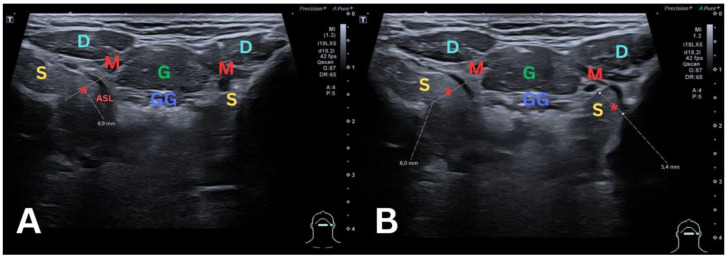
To clarify the changes, an ultrasound examination was performed. In the first image (A), the ultrasound examination, performed with a Canon Aplio i800 device, reveals a defect in the right mylohyoid muscle (M) measuring approximately 9 mm. This defect is associated with a protrusion of the right sublingual salivary gland (S), along with a protrusion of the right sublingual artery (ASL) through the muscle defect (*). In the second image (B), there is an observation of a defect (*) in the left mylohyoid muscle (M) measuring approximately 5 mm, accompanied by a small protrusion of the left sublingual salivary gland (S). These findings indicate significant anatomical changes in the sublingual region, highlighting the presence of muscle defects and glandular protrusions on both sides, which confirmed the diagnosis. S—sublingual gland; M—mylohyoid muscle; *—mylohyoid muscle defect; GG—genioglossus muscle; G—geniohyoid muscle; D—digastric muscle anterior belly; ASL—sublingual artery.
